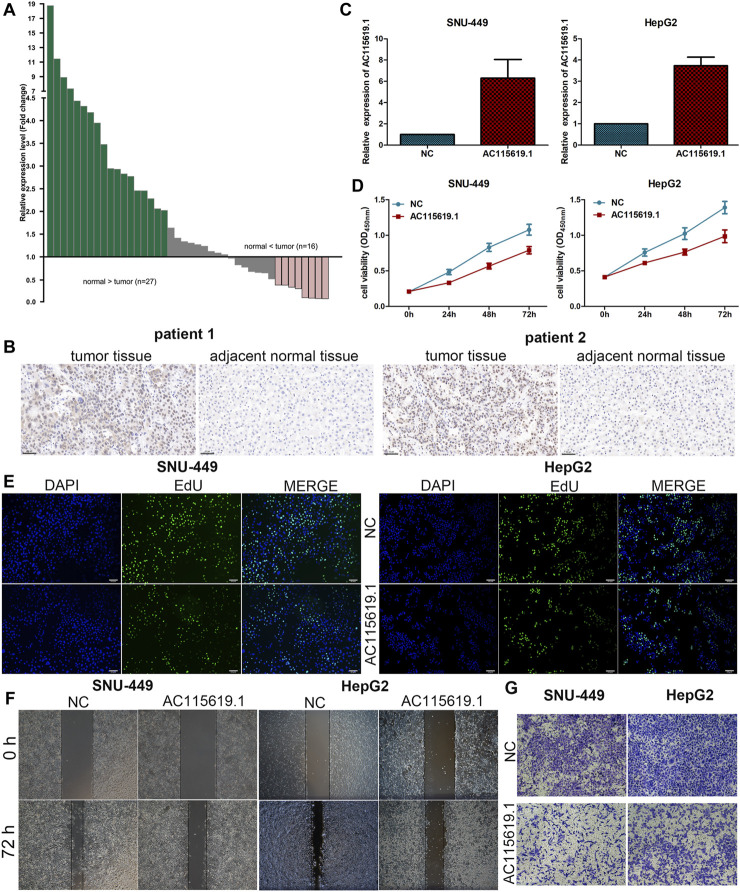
AC115619.1 was negatively related to RBMX and inhibited the progression of HCC. (A) Downregulation of AC115619.1 was validated by qRT-PCR in local HCC samples. Fold changes are analyzed using the formula, 2^- (△△CT (tumor/adjacent normal tissue))^. (B) Representative IHC pictures of RBMX expression in HCC tumor and adjacent normal liver tissues (original magnification: ×200). (C) Transfected efficiency of the AC115619.1 plasmid in HCC cells detected by qRT-PCR. (D) Cell viability of HCC cells after AC115619.1 overexpression detected by CCK8 assay. (E) EdU assay displayed the proliferating ability of HCC cells. (F) Migration ability determined by wound healing assay. (G) Invasion ability determined by transwell assay.
